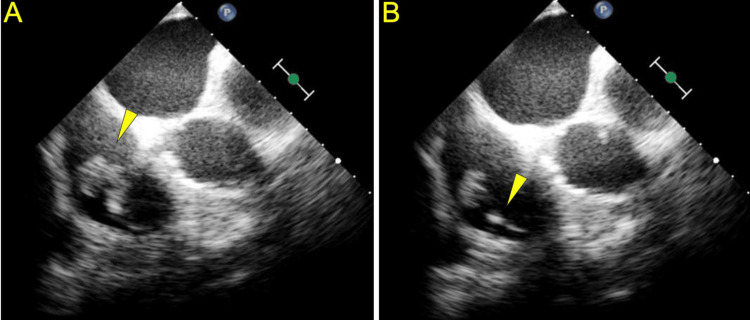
Transesophageal echocardiogram (A) Vegetation on the septal tricuspid leaflet. (B) Vegetation on the pacemaker lead in the right ventricle.
